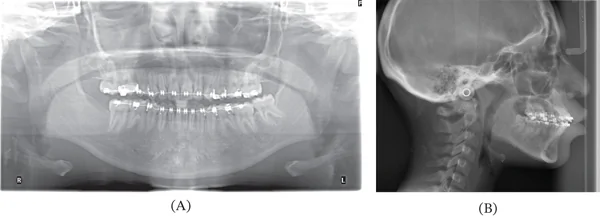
(A) Case #1 panoramic radiograph long‐term follow‐up. (B) Case #1 lateral cephalogram long‐term follow‐up.
